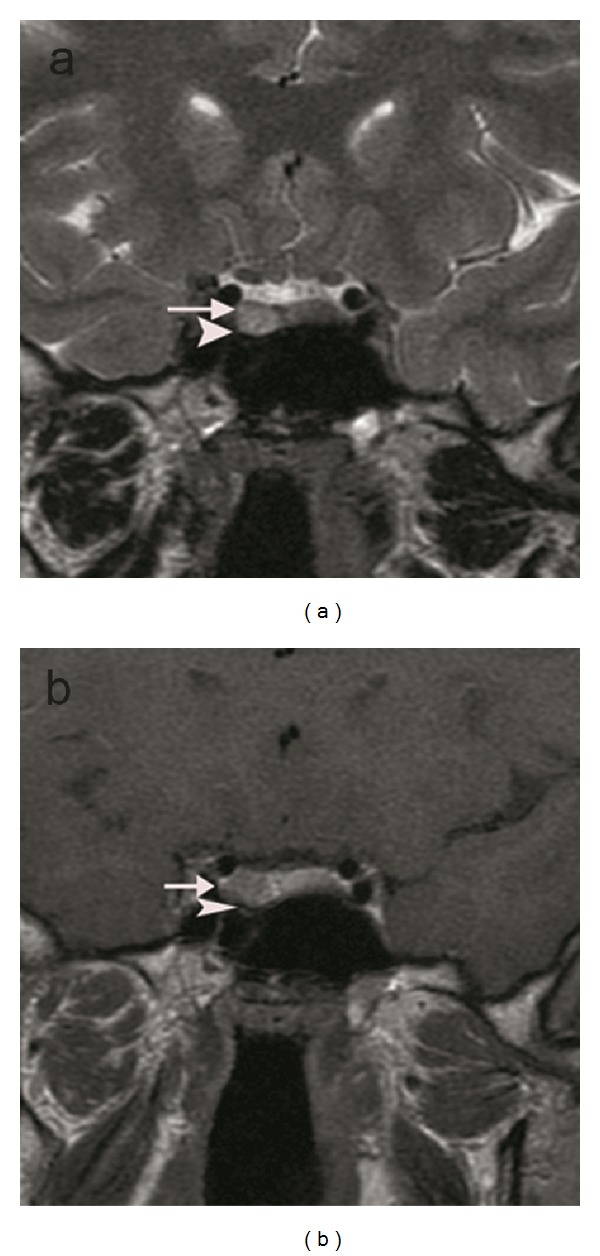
A microadenoma with hyperintensity on T2-weighted coronal (a) and hyperintensity on the T1-weighted coronal image enhanced with IV-GBCM (b) (arrows) on the right side of the gland is shown. Sellar floor ballooning (arrow heads) can be seen on both images.
